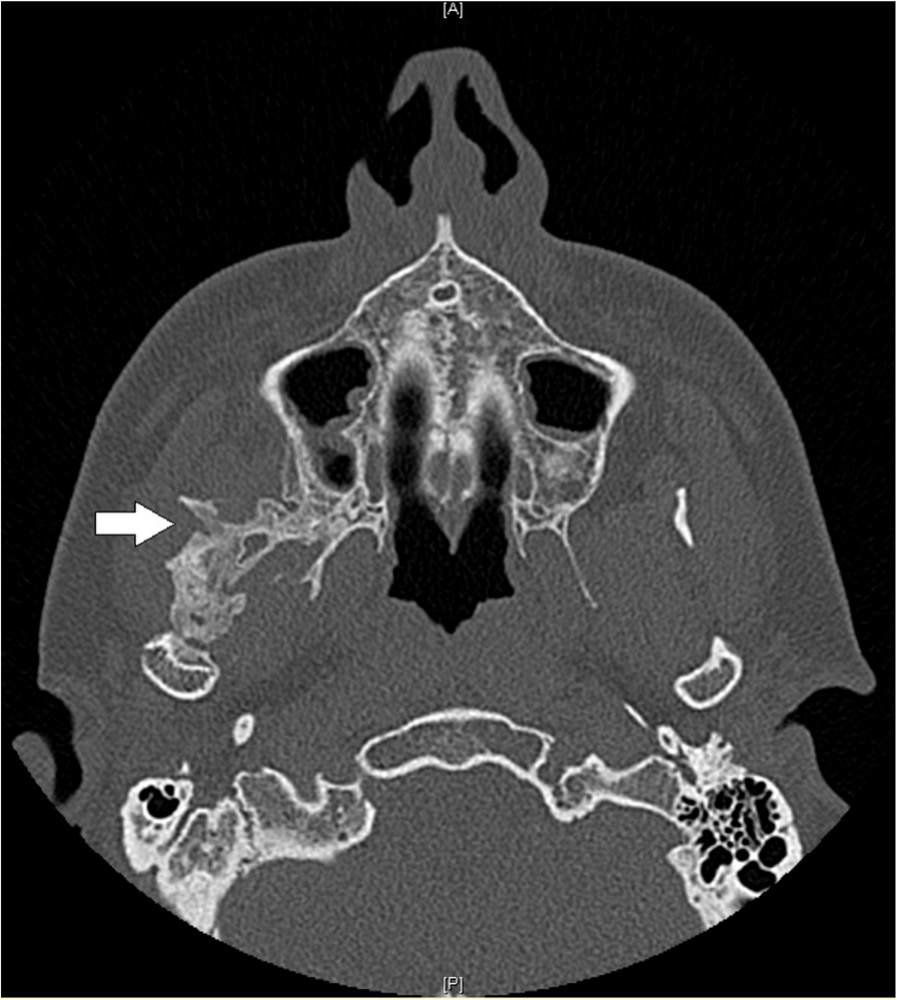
Cone beam scan showing calcification of the right medial pterygoid
